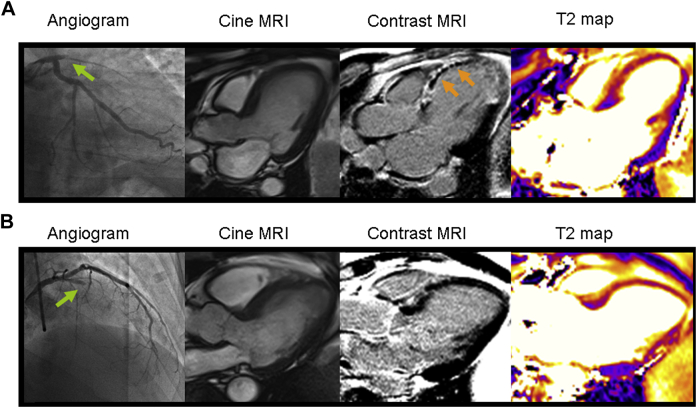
Two Patients With Acute Anterior STEMI One patient was treated by conventional primary PCI with immediate stenting and the other was treated with initial aspiration thrombectomy and then deferred stenting. Each patient had similar ischemic times (147 min and 163 min), and both were treated with similar antithrombotic therapies including 300 mg aspirin, 600 mg clopidogrel, 5,000 IU heparin, and intravenous tirofiban. (A) Usual care with immediate stenting. The angiogram (left) revealed proximal occlusion of the left anterior descending artery (green arrow, Thrombolysis In Myocardial Infarction [TIMI] grade 0 flow). Primary PCI was complicated by no-reflow (TIMI grade 1 flow). Two days later, cardiac MRI was performed. Cine MRI (middle left) revealed an extensive anteroapical left ventricular wall motion abnormality. Matched diastolic phase images obtained using late gadolinium enhancement imaging (middle right) revealed a transmural infarction with microvascular obstruction (orange arrows). The area at risk revealed by T2-weighted imaging (right) was 42.2% of left ventricular mass, and the acute infarct size revealed by late gadolinium enhancement (middle right) was 39.6%. Microvascular obstruction depicted as the central dark zone within the infarct territory was 8.2% of the left ventricular mass. The left ventricular ejection fraction and end-systolic volume were 43.7% and 52.3 ml/m^2^, respectively. Six-month follow-up MRI revealed that the final infarct size was 32.1% of the left ventricular mass, and therefore myocardial salvage was 10.1%. (B) Deferred PCI. The angiogram (left) revealed a proximal occlusion of the left anterior descending artery (green arrow, TIMI grade 0 flow). Primary PCI in the left anterior descending coronary artery with deferred stent implantation was uncomplicated. Two days later, cardiac MRI was performed. Cine MRI (middle left) revealed an extensive anteroapical left ventricular wall motion abnormality. Despite similar ischemic times in both patients, this patient had minimal evidence of infarction on late gadolinium enhancement imaging (middle right) and no microvascular obstruction. The acute infarct size was 8.4% of the left ventricular mass. The area at risk (right) was similar between cases, at 43.1%, as was the left ventricular ejection fraction and normalized end-systolic volume, measured at 43.5% and 57.1 ml/m^2^, respectively, consistent with stunned but viable myocardium. Six-month follow-up MRI revealed that the final infarct size was 1.7% of the left ventricular mass, and therefore myocardial salvage was 41.4%.
